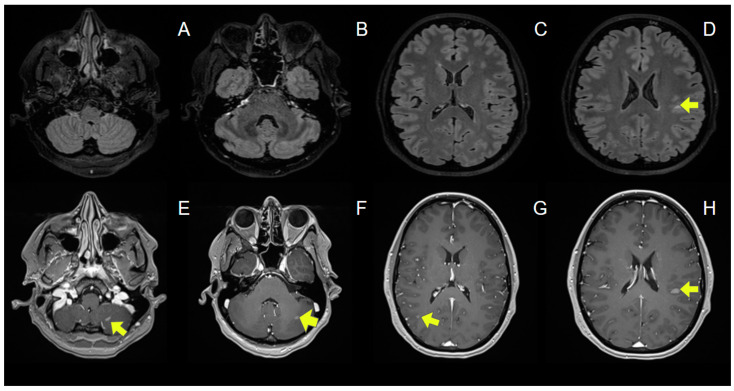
Brain MRI prior to radiation therapy. Metastatic lesions in the gray–white matter junction are indicated by arrows on axial FLAIR (D) and axial post-gadolinium T1-MPRAGE images (E–H). (A–H). Abbreviations: FLAIR: fluid-attenuated inversion recover; MPRAGE: magnetization prepared rapid gradient echo.
